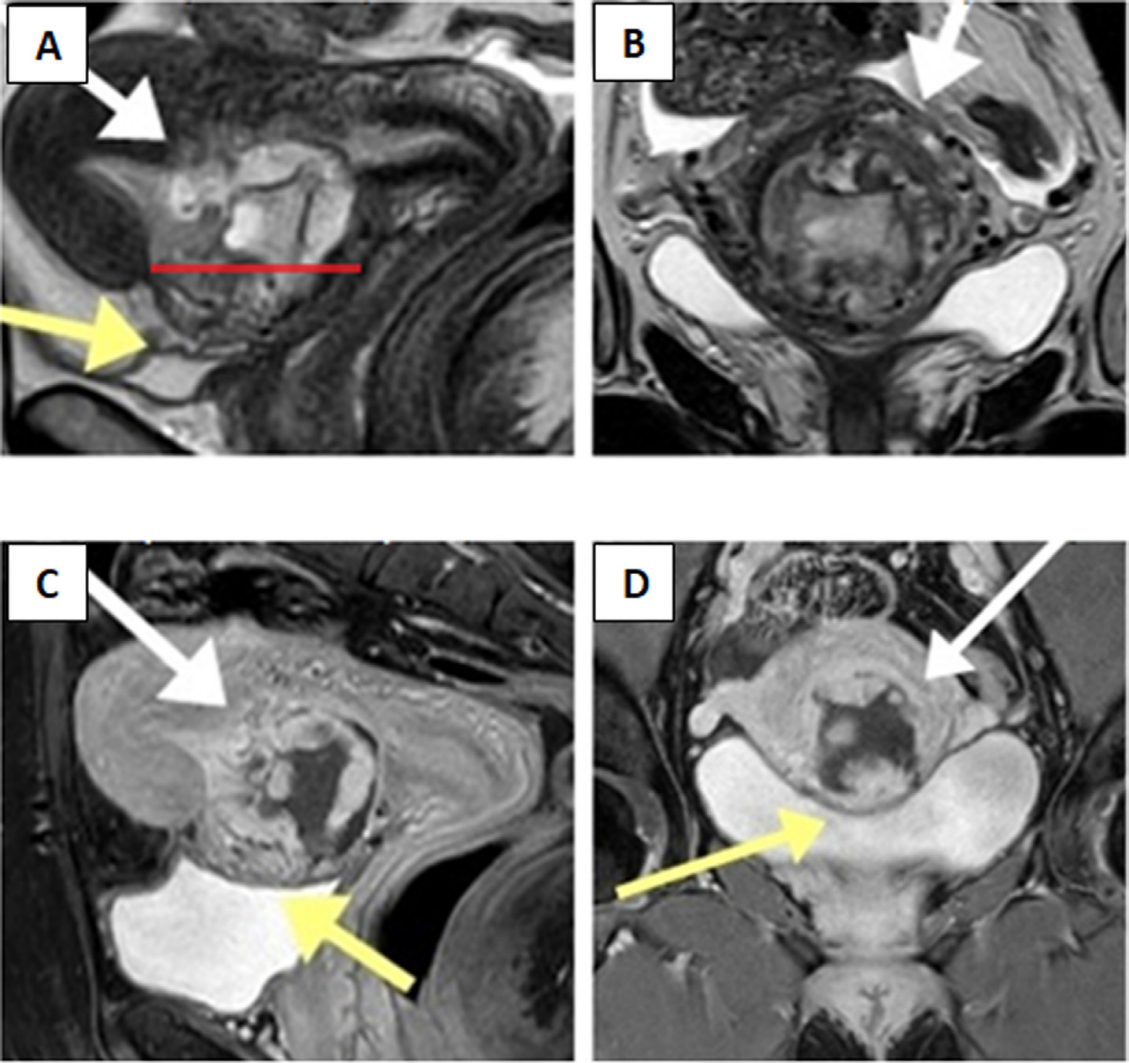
(A) Sagittal T2-weighted magnetic resonance image demonstrates gestational sac as heterogeneous mass in the anterior part of lower uterine wall within the prior caesarean scar site, extending into the uterine cavity (white arrow). The scar margins are separated (red line) following infiltration by ectopic tissue. The “tenting sign’’ (yellow arrow) suggests trophoblast invasion into the wall of urinary bladder. (B) Coronal T2-weighted sequence demonstrates the disruption of thin myometrium secondary to pathological infiltration (white arrows). (C, D) Sagittal and coronal T1-weighted delayed post-contrast magnetic resonance images with full urinary bladder show no “tenting sign’’ (yellow arrows) evident in A image, therefore transmural trophoblast growth into the urinary bladder can be excluded
